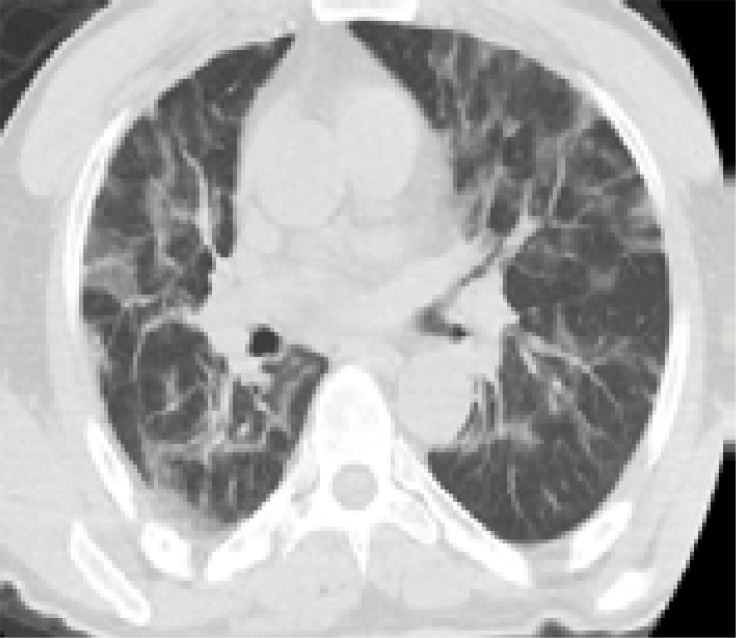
E) A 57-year old female patient applied to hospital with complaints of fatigue and cough for 2 weeks. Fibroatelectatic line in the lower lobe of right lung and nodular ground glass density (black arrows) were found in chest CT image of the patient.
